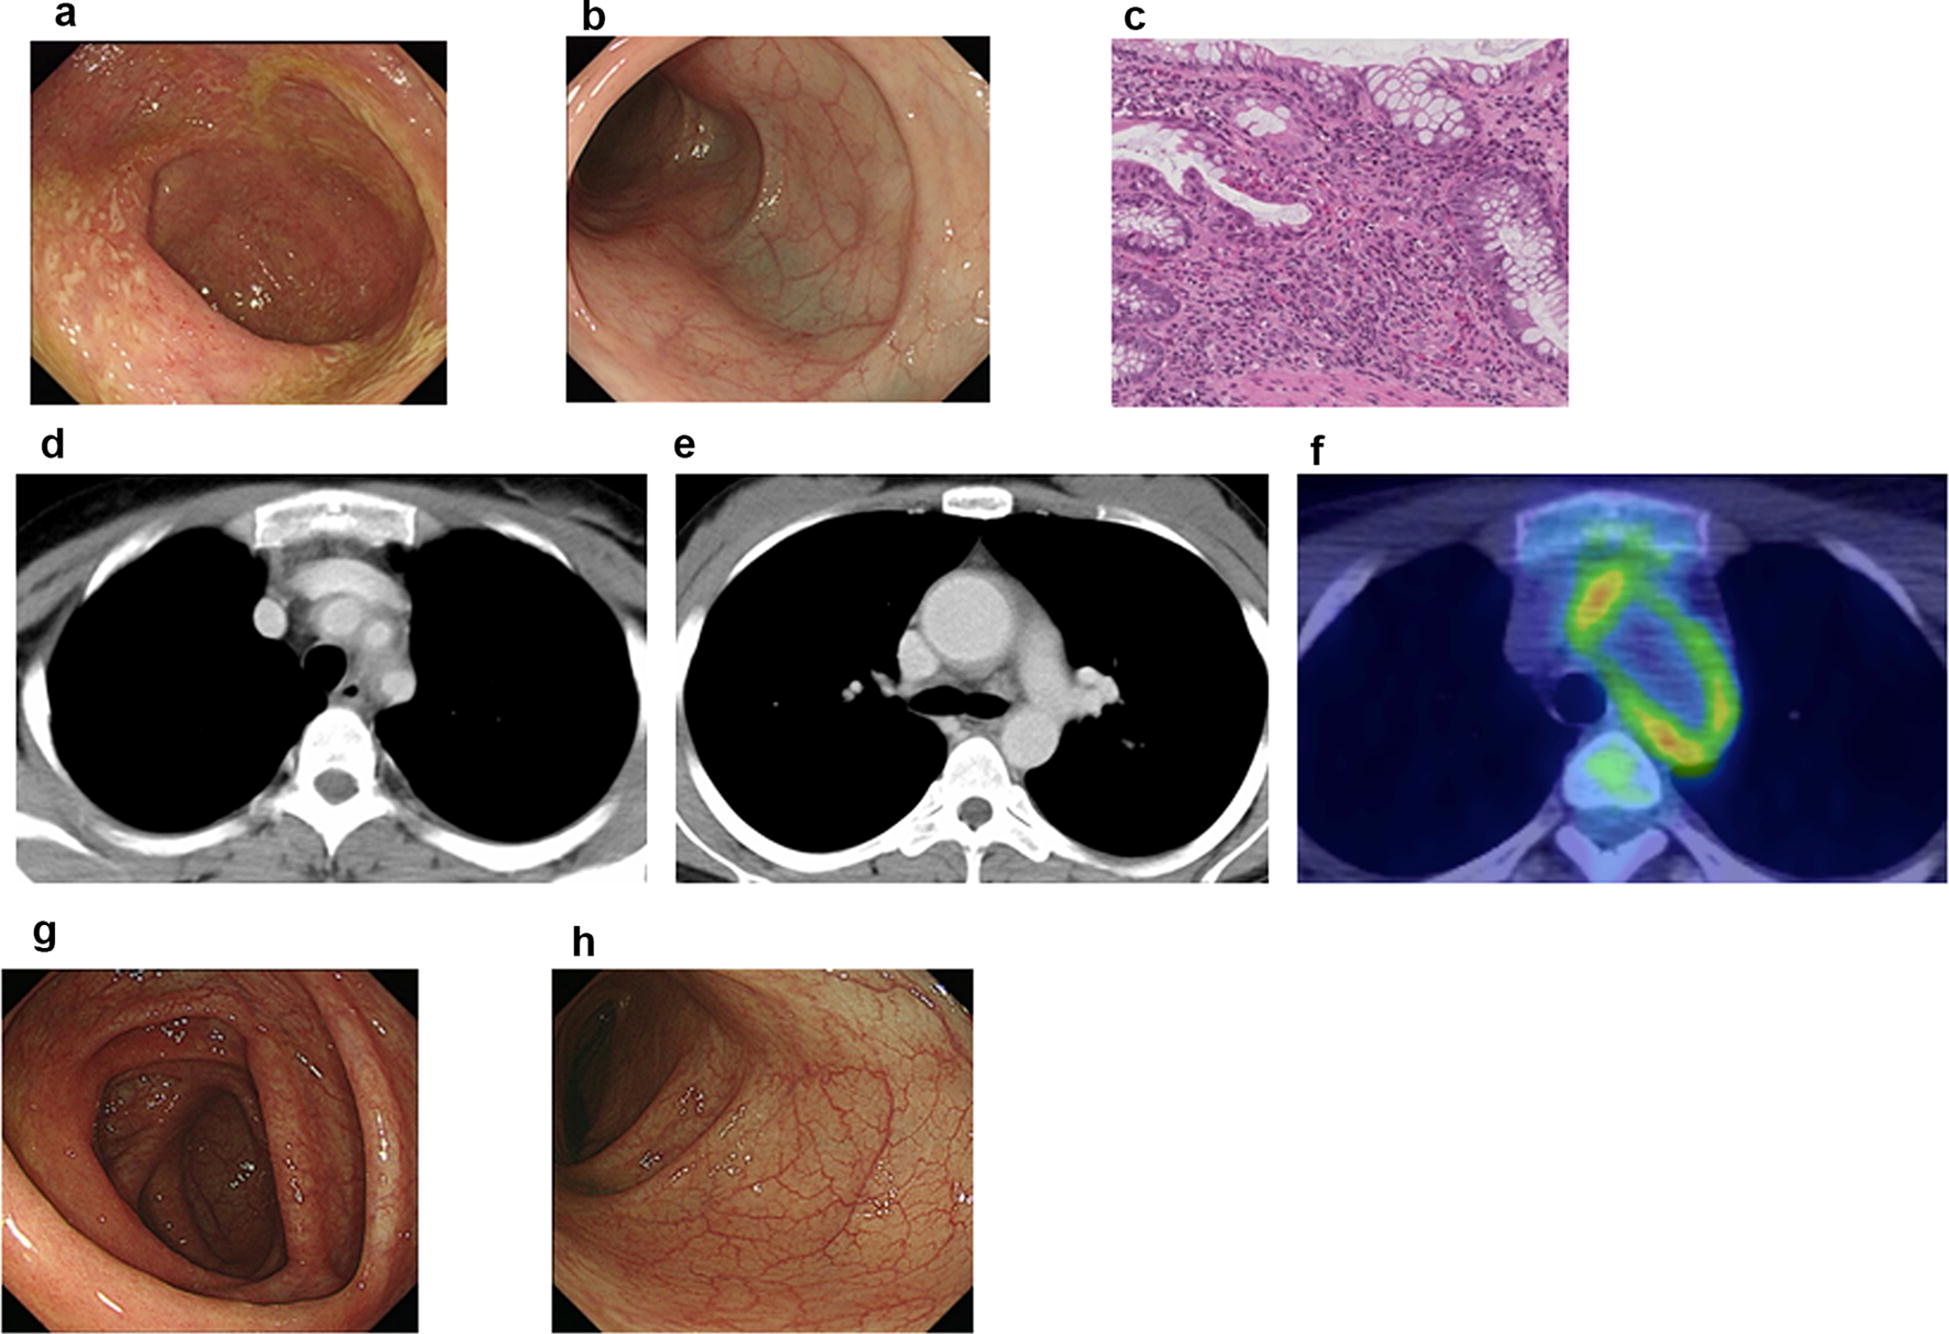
Endoscopic findings of the colon (a cecum, b sigmoid colon) and pathological findings of the sigmoid colon (c) when the case was diagnosed as EGE. Computed tomography scan showing wall thickening of the aortic arch, brachiocephalic artery, right and left subclavian artery and left common carotid artery as well as dilation of the ascending aorta (d, e). Positron emission tomography (PET) scans showing the FDG uptake along the aortic arch, brachiocephalic artery, right and left subclavian artery and left common carotid artery (f). Endoscopic findings of the colon (g cecum, h sigmoid colon) when the case was diagnosed to have TA
